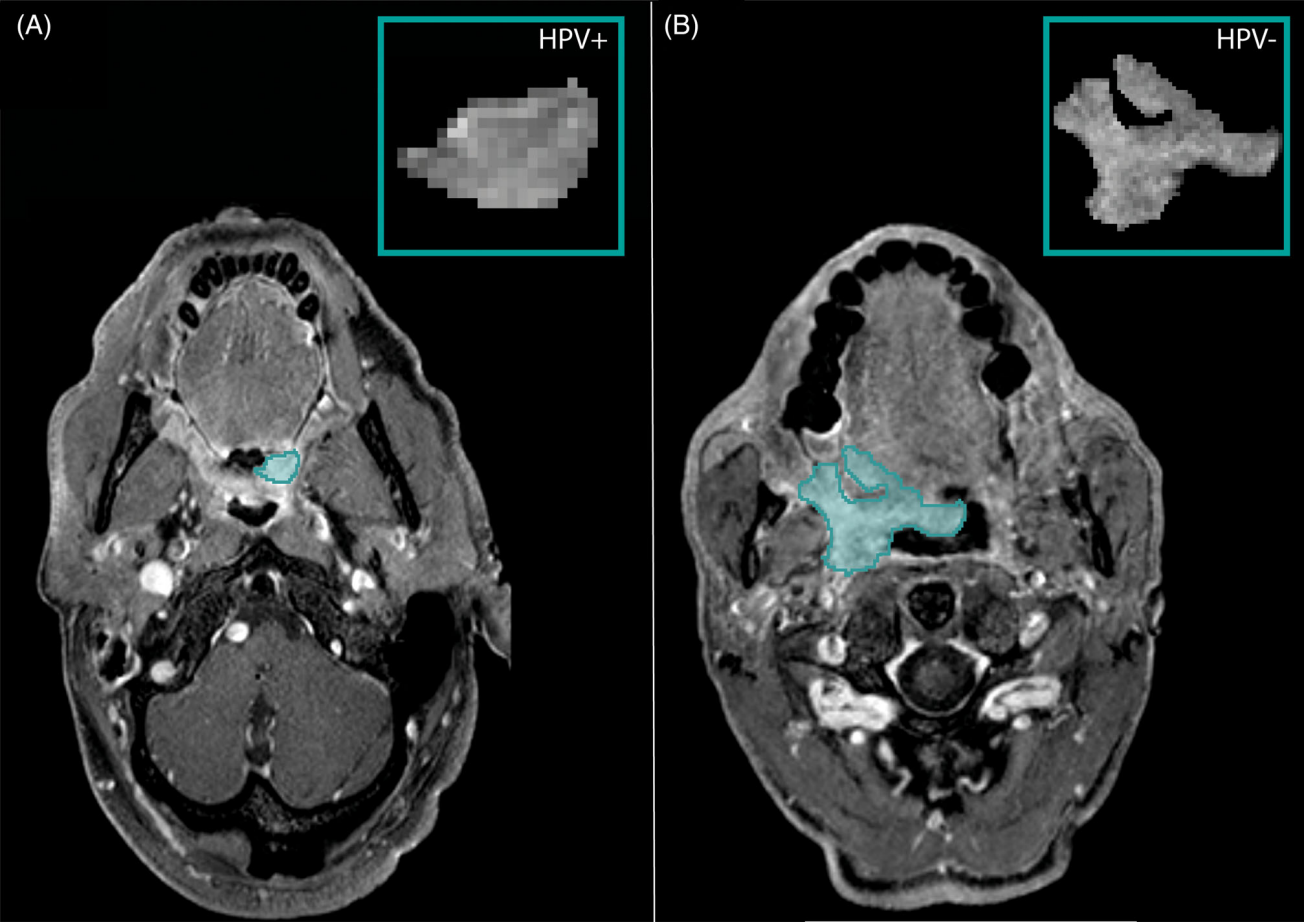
Magnetic resonance image of a patient with human papillomavirus (HPV)‐positive (A) and HPV‐negative (B) tumor status (blue marked area) showing difference in textural appearances. The patient with a HPV‐positive tumor status has a smaller and rounder tumor. Intensity values were less variated and less changes of intensities were visible [Color figure can be viewed at wileyonlinelibrary.com]
